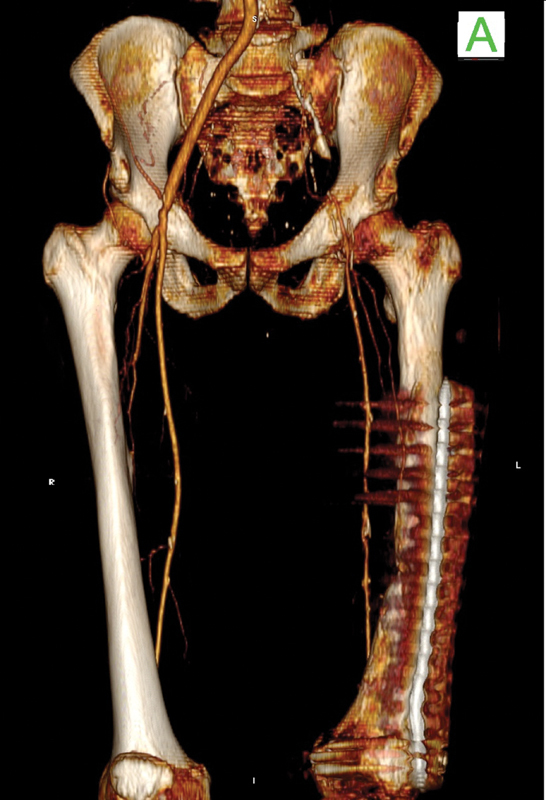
Preoperative three-dimensional computed tomography scan reconstruction. The anterior view shows the thrombosed left limb of the polyethylene terephthalate graft with sufficient ipsilateral distal perfusion.
